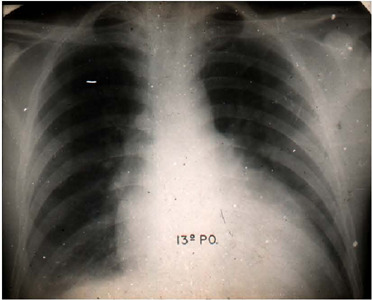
Radiography of João “boiadeiro” at the 13^th^ postoperative
